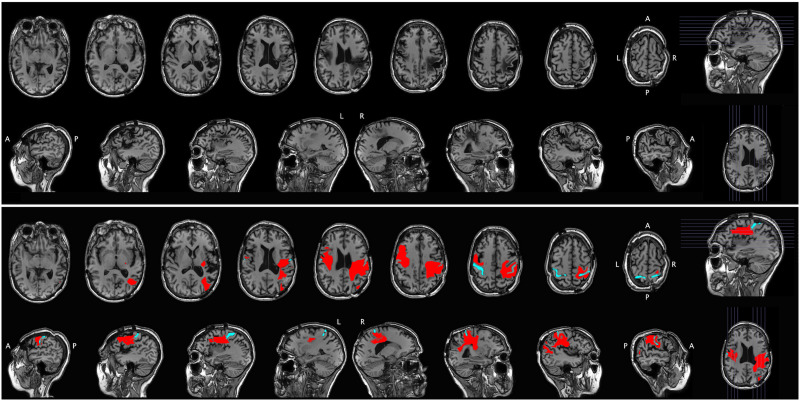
Structural MRI for Case 2. The raw scan is shown in the top portion of the figure, with axial slices (first row) and sagittal slices (second row). The same scan is shown in the bottom portion of the figure, with the lesion segmentation highlighted in red and the central sulcus highlighted in cyan for reference. A = anterior, P = posterior, L = left, R = right.
